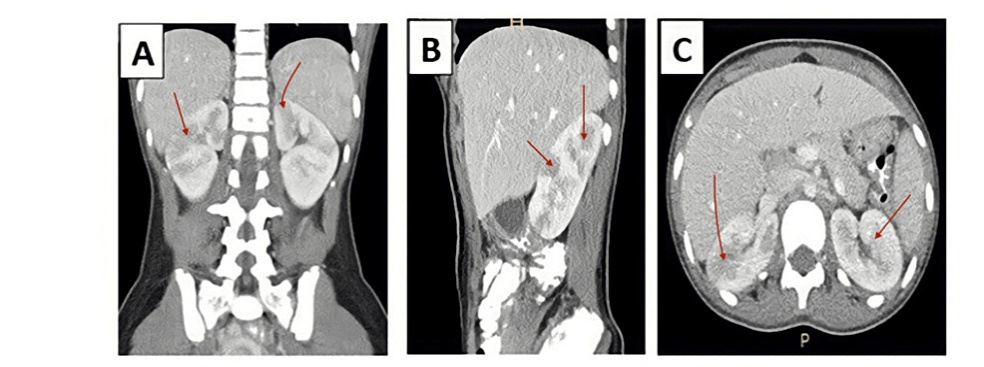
Coronal (A), sagittal (B), and axial (C) views of abdominal computed tomography scan with intravenous contrast. The arrows indicate multiple focal areas of hypoattenuating lesions involving both kidneys, suggestive of bilateral renal infarcts.
